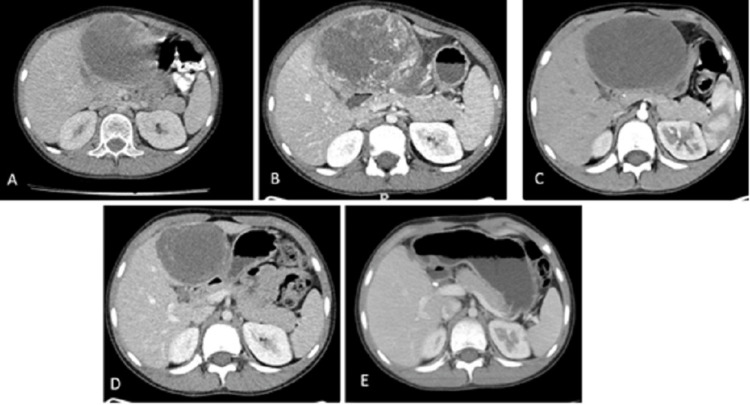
Tomographic evolution in the axial axis of the abdomen. A. Extrainstitutional image at the time of diagnosis: exophytic lesion located in segment IV measuring 87 x 75 mm, partially compressing the gallbladder, contacting its wall without infiltration. B. CT scan performed upon admission to the institution reports a lesion with dimensions of 103 x 85 mm located in segment IV. C. CT scan performed after receiving 2 cycles of ifosfamide/doxorubicin chemotherapy: predominantly liquid mass located in hepatic segment IV, measuring 95 x 69 mm.  D. CT scan performed after 4 cycles of systemic chemotherapy (2 cycles of ifosfamide/doxorubicin and 2 cycles of VAC): Hepatic lesion in segment IV with dimensions of 72 x 60 mm. E. CT scan performed as end-of-treatment evaluation after complete local surgical control and 2 additional cycles of adjuvant chemotherapy: post-surgical changes without evidence of neoplastic involvement.
